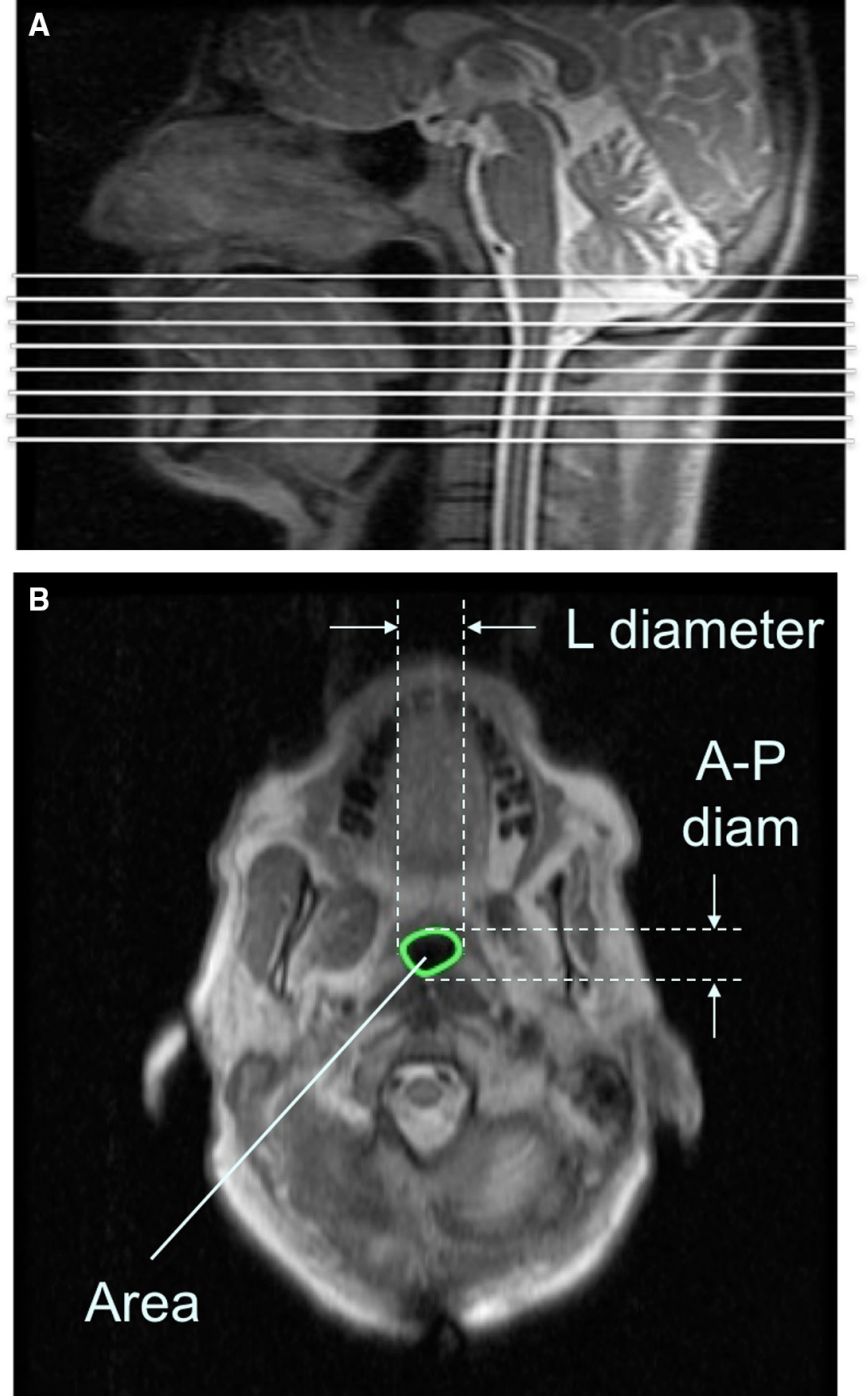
(A) Sagittal image of the upper airway showing the location of the axial slices (white lines) from the hard palate down to the epiglottis. (B) Axial magnetic resonance image of a sample subject (control), demonstrating the upper airway parameters: Area, Lateral (L) and anterior–posterior (A‐P) diameter. Diam: diameter.
